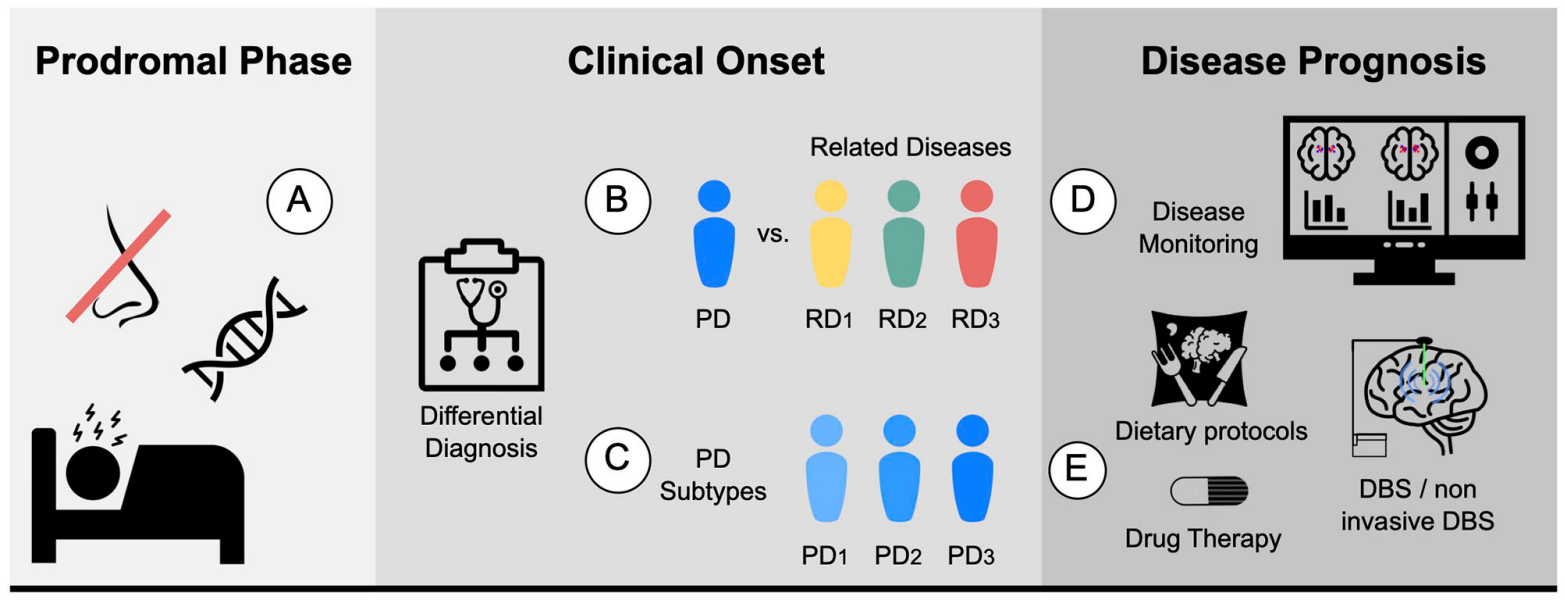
Proposed biomarkers for PD using MRI: (A) Prodromal biomarker: identifying brain changes during the prodromal phase. (B) Differential diagnosis biomarker: assisting in distinguishing PD from related diseases. For instance, ET or MSA. (C) Subtype biomarker: classifying PD patients into their corresponding subtypes. (D) Progression biomarker: aiding in predicting the progression of the disease and treatment response with disease monitoring. (E) Therapy response biomarker: facilitating personalized medicine by finding the best drug, dietary protocols, physical or cognitive therapies, and predicting the potential response to other therapies such as DBS and non-invasive DBS.
